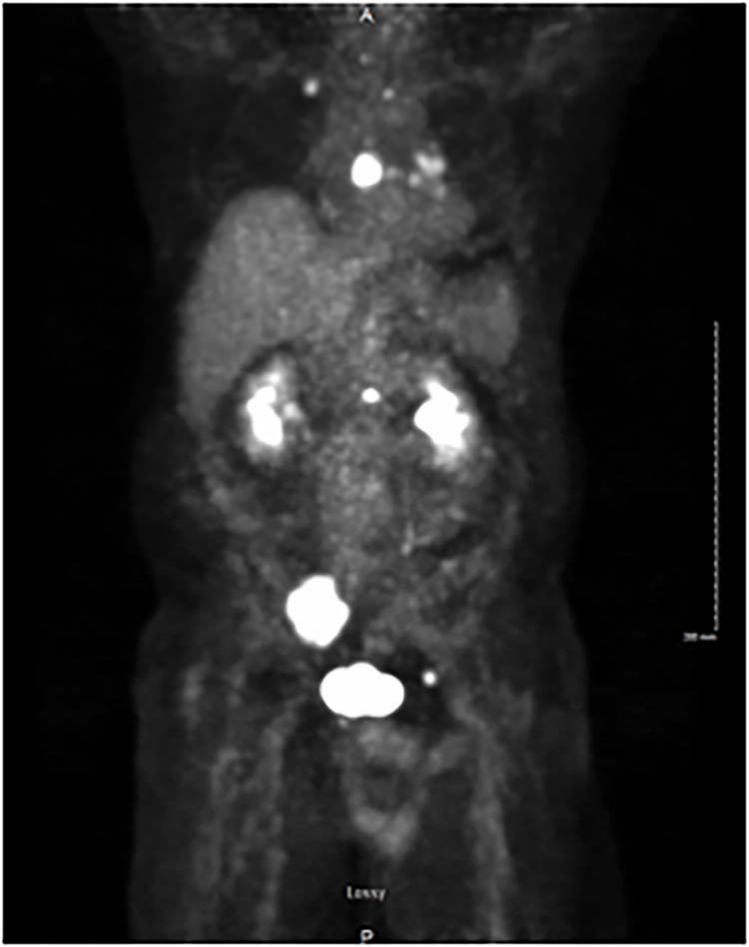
PET/CT demonstrating isotope uptake indicative of metastatic disease. Coronal section demonstrating no evidence of neoplastic disease in the head and neck region. Several foci of metastatic disease are present at the level of thoracic inlet. Shown are a 5 cm lytic metastatic focus involving R sacrum, 1.5 cm isotope positive focus of lytic metastatic disease involving the mid-portion of the sternum, and smaller positive foci involving left side of body of L-1, anterior aspect of left acetabulum, posterior aspect of 7th left rib, medial aspect of right clavicle and left transverse process of T-1.
